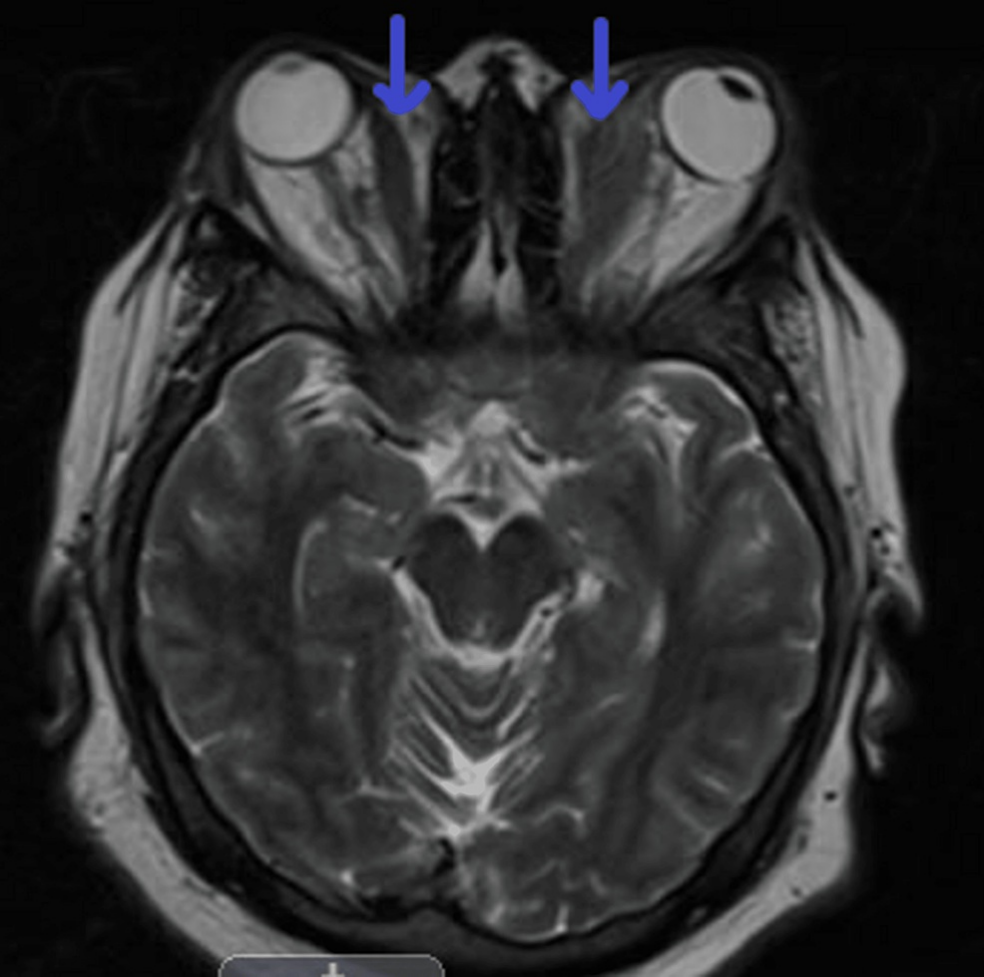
Orbital MRI (T2, axial view) showing significant enlargement of extraocular muscles (bilateral medial recti indicated by blue arrows) MRI: magnetic resonance imaging
